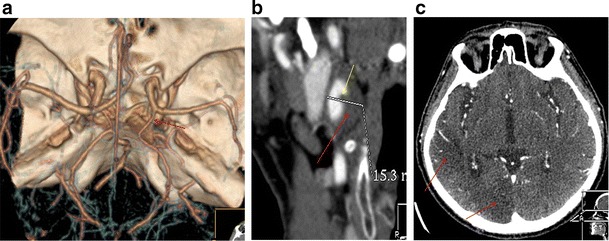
a CTA (VRT reconstructions) shows a right-sided foetal Pcom (*red arrow*). b CT oblique MPR image shows an increased wall thickness (*red arrow*) and intimal flap (*yellow arrow*) of the dissected right ICA. c Axial CT image at the level of the third ventricle shows right-sided occipital and posterior parietal lobe infarcts (*red arrows*)
